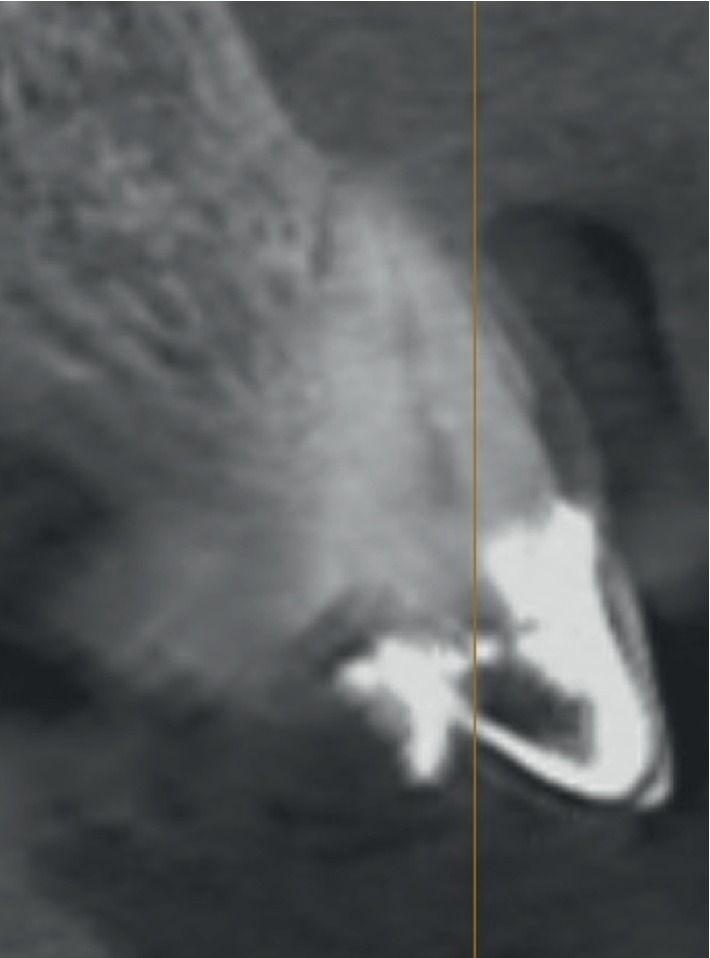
Case 1: initial CBCT for evaluation of the cross section of element 2.1 with a root fracture.
